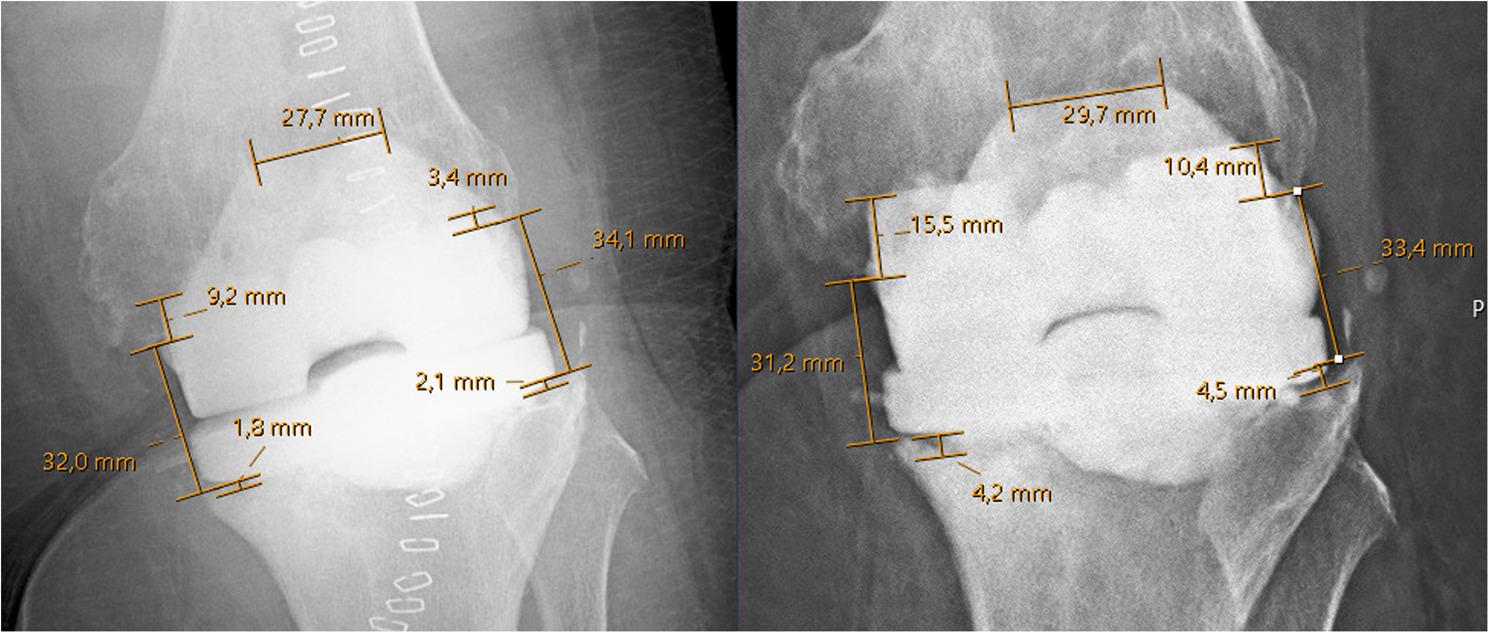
Radiographic assessment of femoral and tibial bone loss using standardized cortical reference points on anteroposterior knee radiographs. Measurements were performed by calculating the distance between defined cortical landmarks of the femur and tibia and the spacer interface. The same anatomical reference points were consistently used at both time points (immediately after spacer placement and prior to reimplantation) to ensure reproducibility and comparability of measurements
